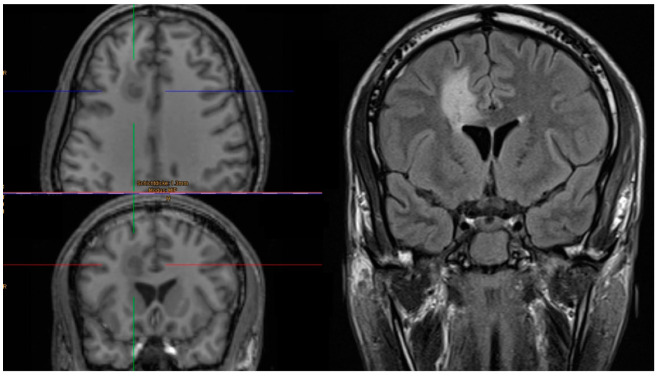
Axial T1-weighted MRI with corresponding coronal imaging showing a lesion in right frontal lobe. Coronal FLAIR demonstrating local edema. The patient underwent 3 MRI examinations with an ICP probe in situ.
